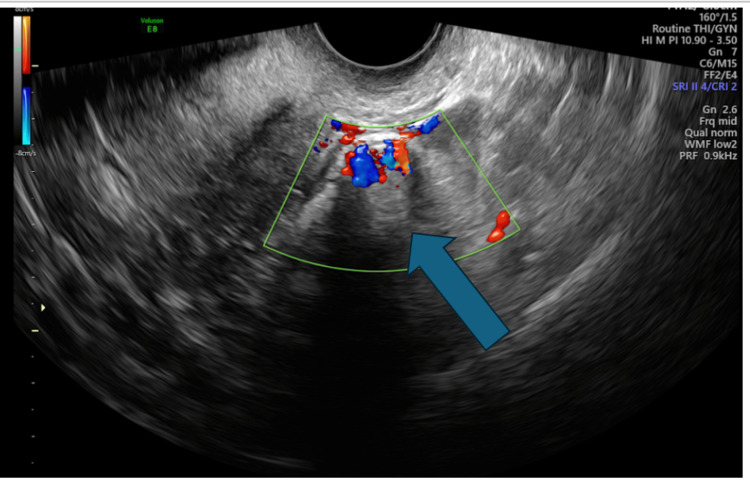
Transvaginal ultrasound (mode Doppler): Vascularity is seen within the possible ectopic pregnancy (blue arrow).
